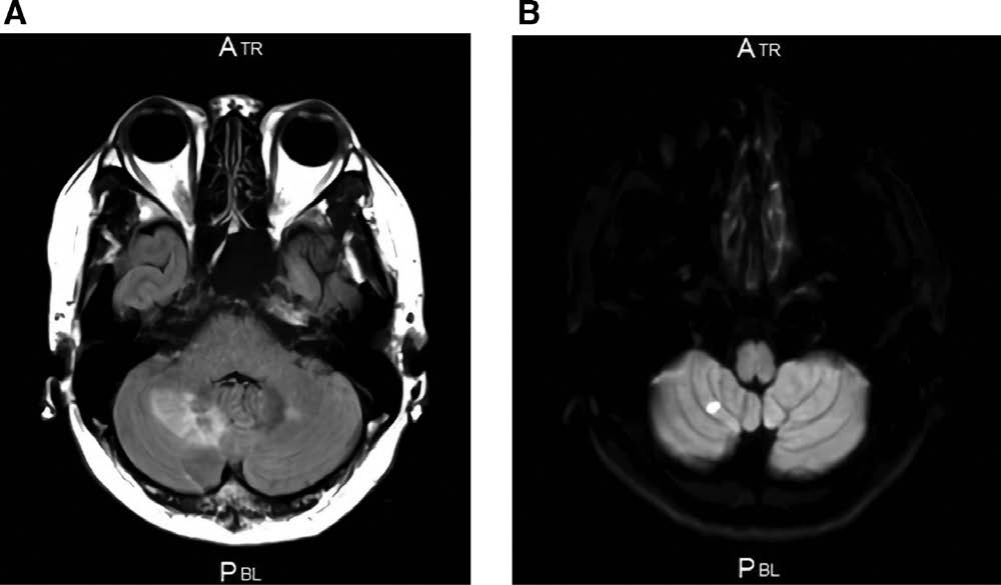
(A) Brain magnetic resonance imaging showing hyperintense lesions on fluid-attenuated inversion recovery in the white matter of both cerebral and right cerebellar hemispheres. (B) Hyperintense lesion on diffusion imaging in the right cerebellar hemisphere.
